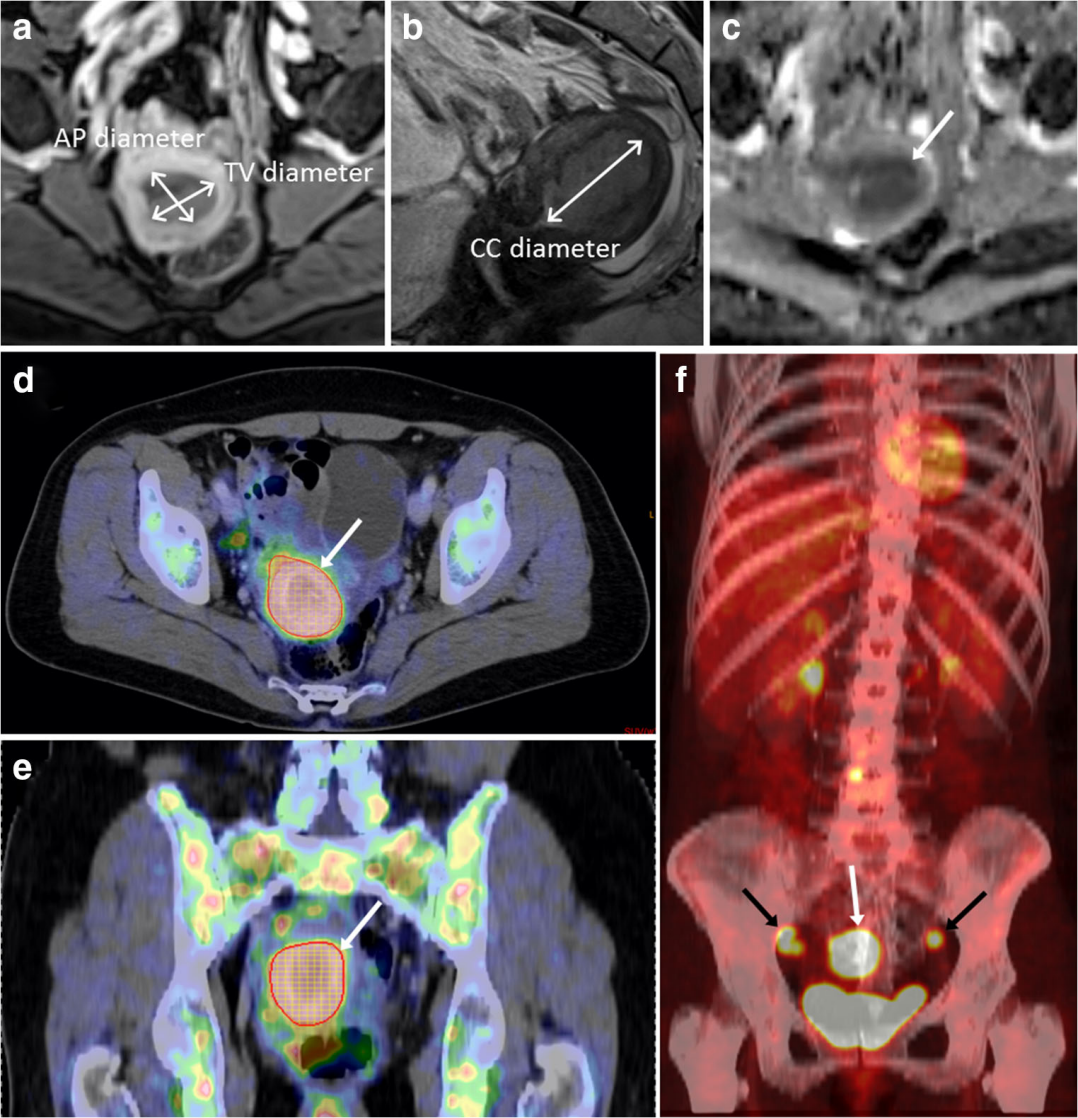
Pelvic MRI (a–c) and whole-body 18F-FDG PET/CT (d–f) of a 60-year-old patient with endometrial carcinoma, FIGO stage IIIC (endometrioid, grade 3). a Axial oblique contrast-enhanced T1-weighted MRI and (b) sagittal T2-weighted MRI for measurement of maximum tumor diameters in three orthogonal planes (AP, TV, and CC diameters). c On apparent diffusion coefficient map, the tumor (arrow) is depicted as hypointense indicating restricted diffusion. d Axial and (e) frontal 18F-FDG PET/CT show the metabolic tumor volume (MTV) (red line shows the MTV boundaries) in which the maximum and mean standardized uptake values are measured. f Maximum intensity projection map depicts the 18F-FDG-avid primary tumor (white arrow) and 18F-FDG-avid pelvic metastatic lymph nodes (black arrows)
